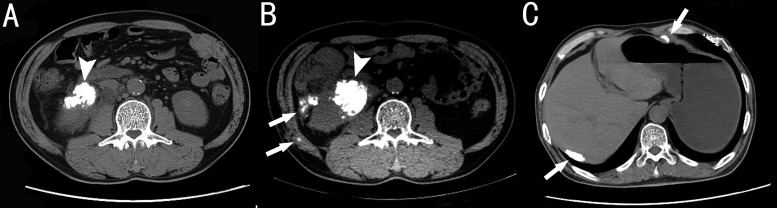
Representative abdominal computed tomography (CT) findings (A) prior to and (B and C) following the first surgery. (A) Prior to the first surgery, abdominal CT showed a large dense mass with calcification (arrowhead) located below the right kidney. (B) Two months after the first surgery, CT demonstrated a mass (arrowhead), with a large calcified lesion, anterior to the right psoas muscle. The renal fascia and abdominal wall showed multiple calcified foci (arrow). (C) Nine months after the first surgery, CT revealed calcified lesions (arrow) in the outer stomach anterior wall and hepatic surface.
